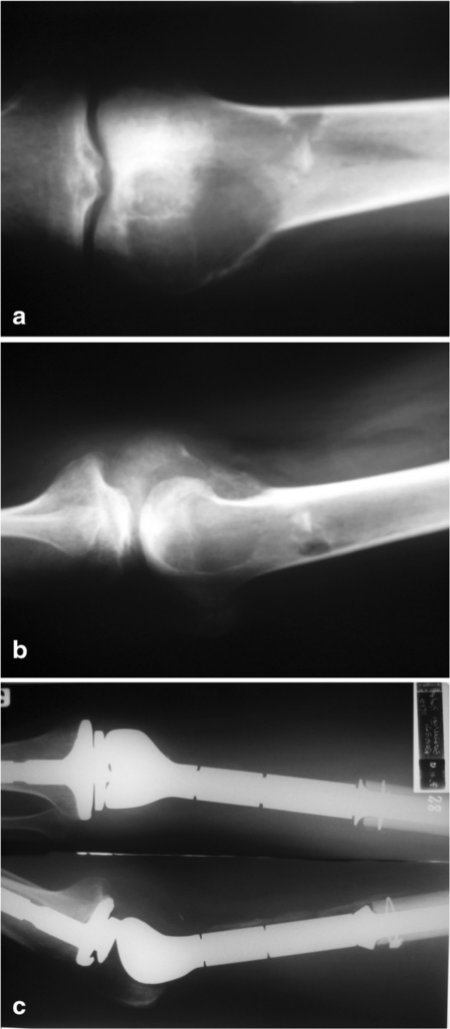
Typical indications for modular endoprosthetic replacement in the distal femur. Bone destruction by metastatic breast carcinoma (a, b) and radiograph of the modular endoprosthetic replacement (c)
